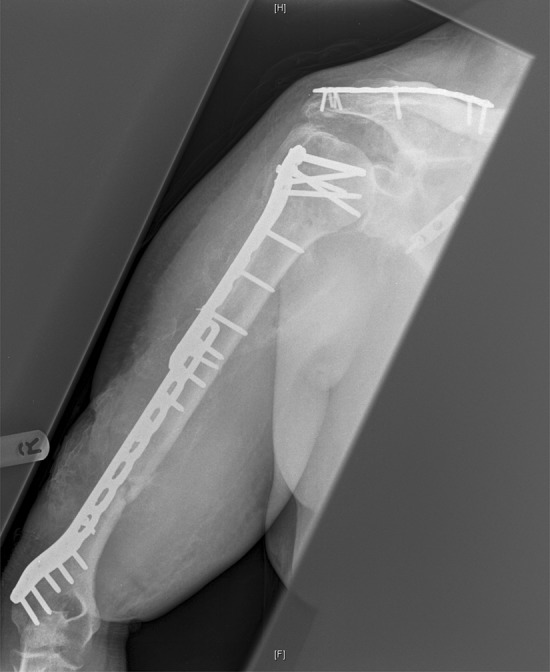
AP radiograph of the humeral fracture at 41 days after presentation
